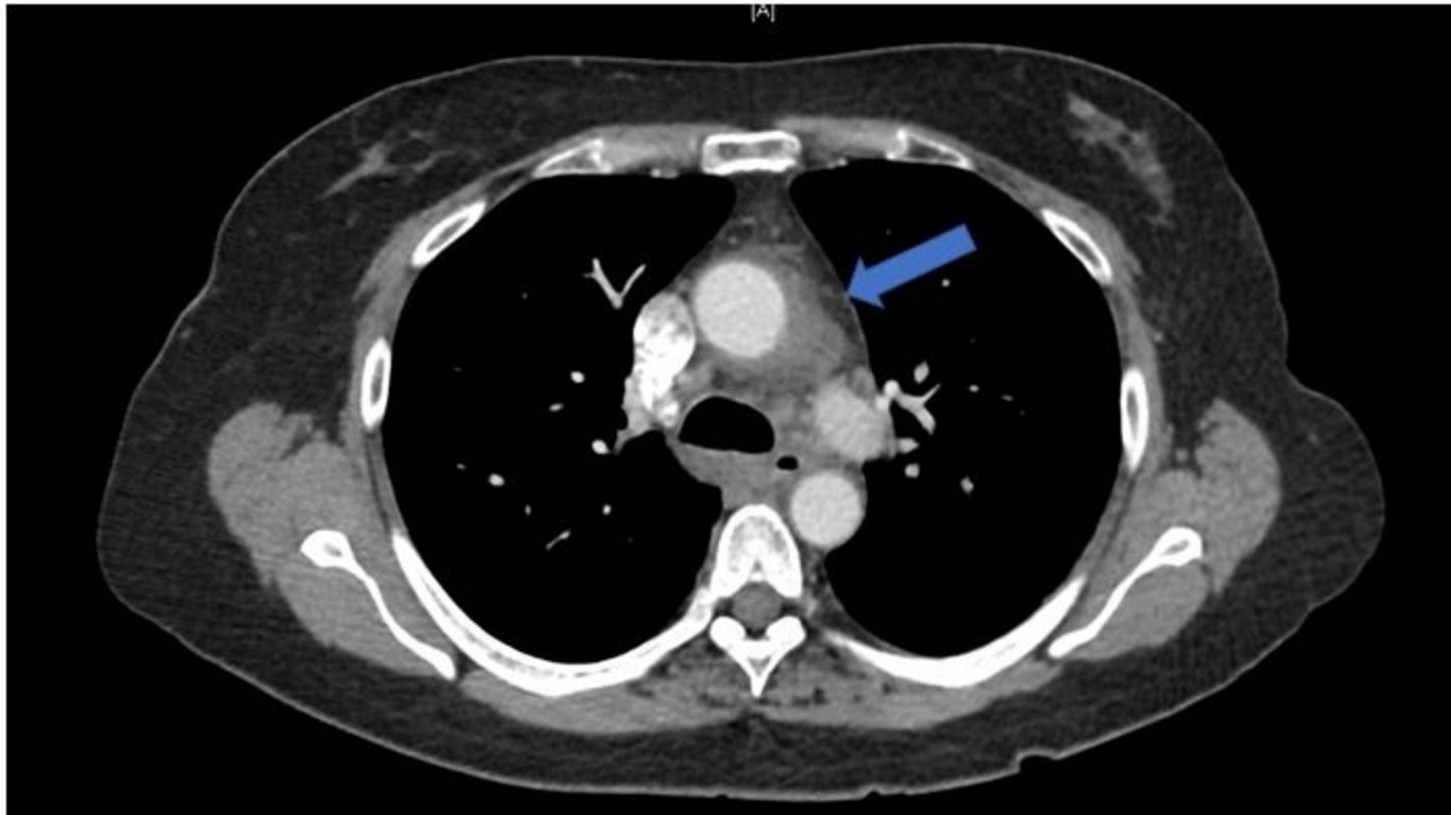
Aortitis on CT chest Computed tomography (CT) chest without contrast obtained during the March 2018 admission for fevers and constitutional symptoms. Diffuse fat stranding is seen surrounding the ascending aorta and aortic arch (arrow), consistent with aortitis.
